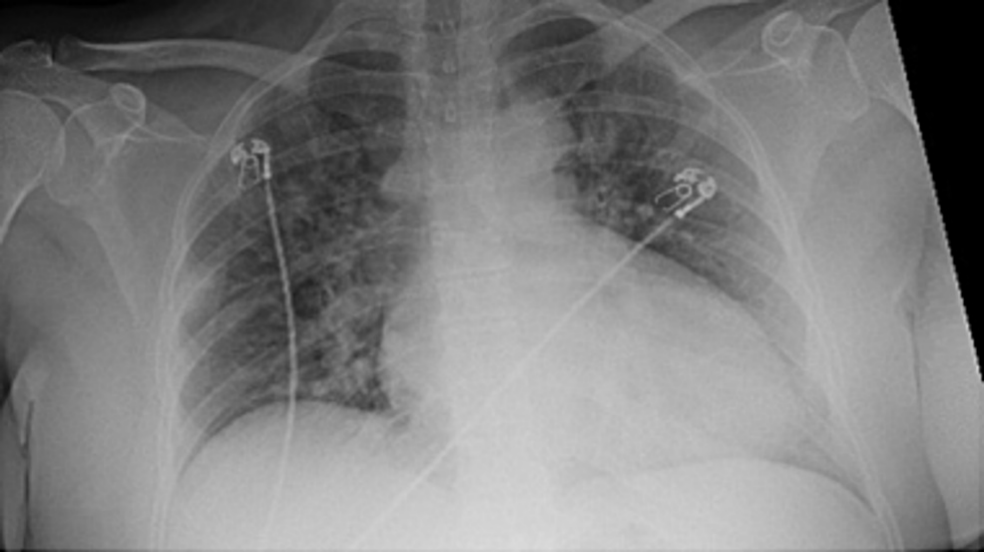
Chest X-ray The imaging shows bilateral small infiltrates consistent with COVID-19. There is no sign of cardiomegaly COVID-19: coronavirus disease 2019
